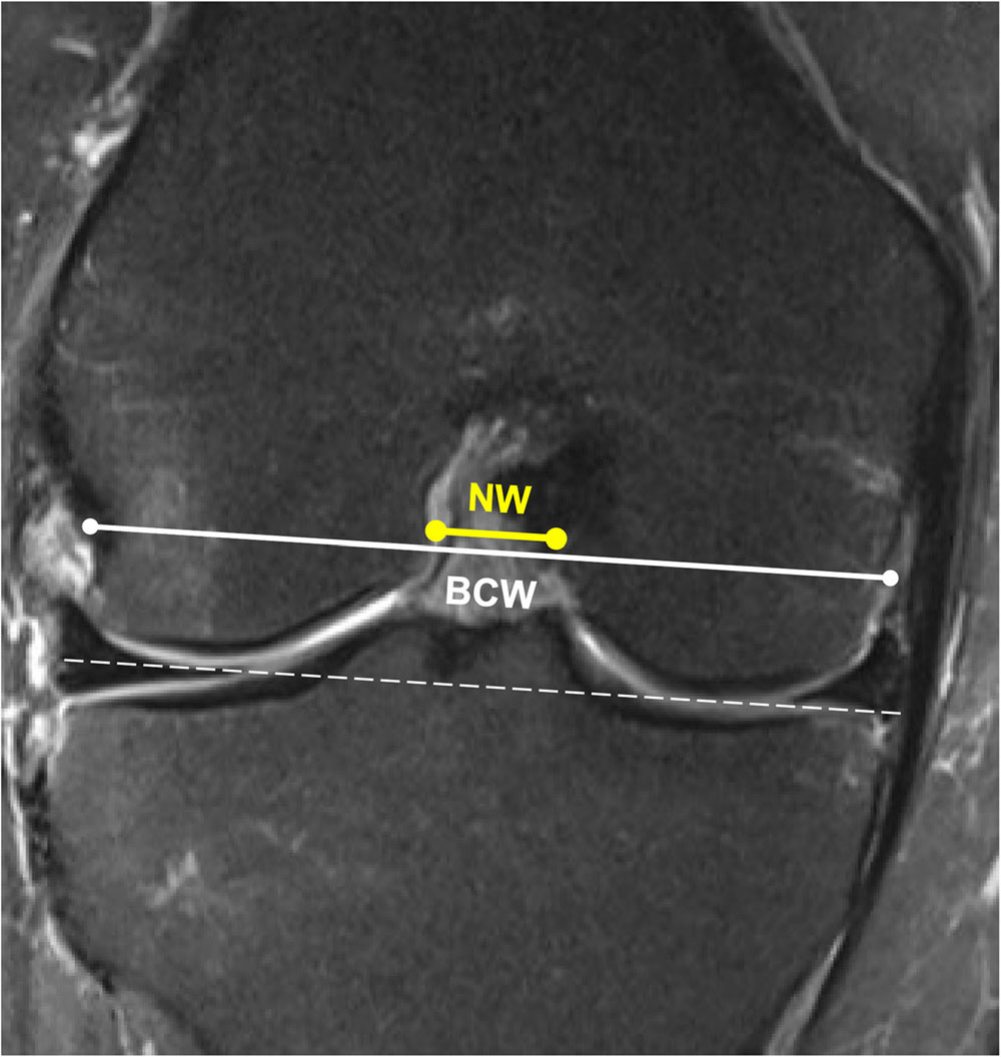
Measurement of the intercondylar notch width (NW) and intercondylar NW index (NWI) on a coronal magnetic resonance image. A line is drawn tangential to the distal aspects of the femoral condyles (dashed white line). The bicondylar width (BCW) is then determined through the superimposition of a parallel line at the intersection of the apex of the popliteus sulcus (white line). The NW (yellow line) corresponds to the width of the intercondylar notch at the level of the BCW. The NWI can subsequently be calculated by dividing the NW with the BCW.
